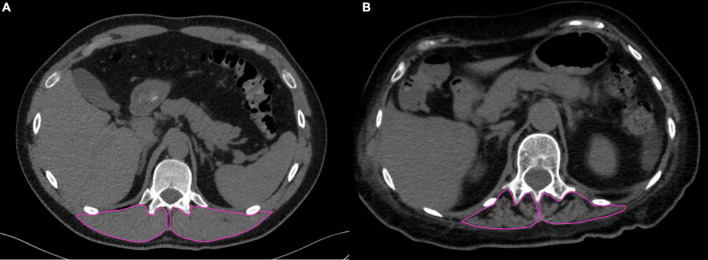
Chest CT images at the T12 level in two patients with COVID-19 pneumonia. In (A) (30-year-old man), the skeletal muscle area segmented at the T12 level is characterized by normal mass and high mean SM-RA (56.5 HU); in (B) (72-year-old woman), impaired muscle status is evident, with fat infiltration and low mean SM-RA (11.1 HU).
